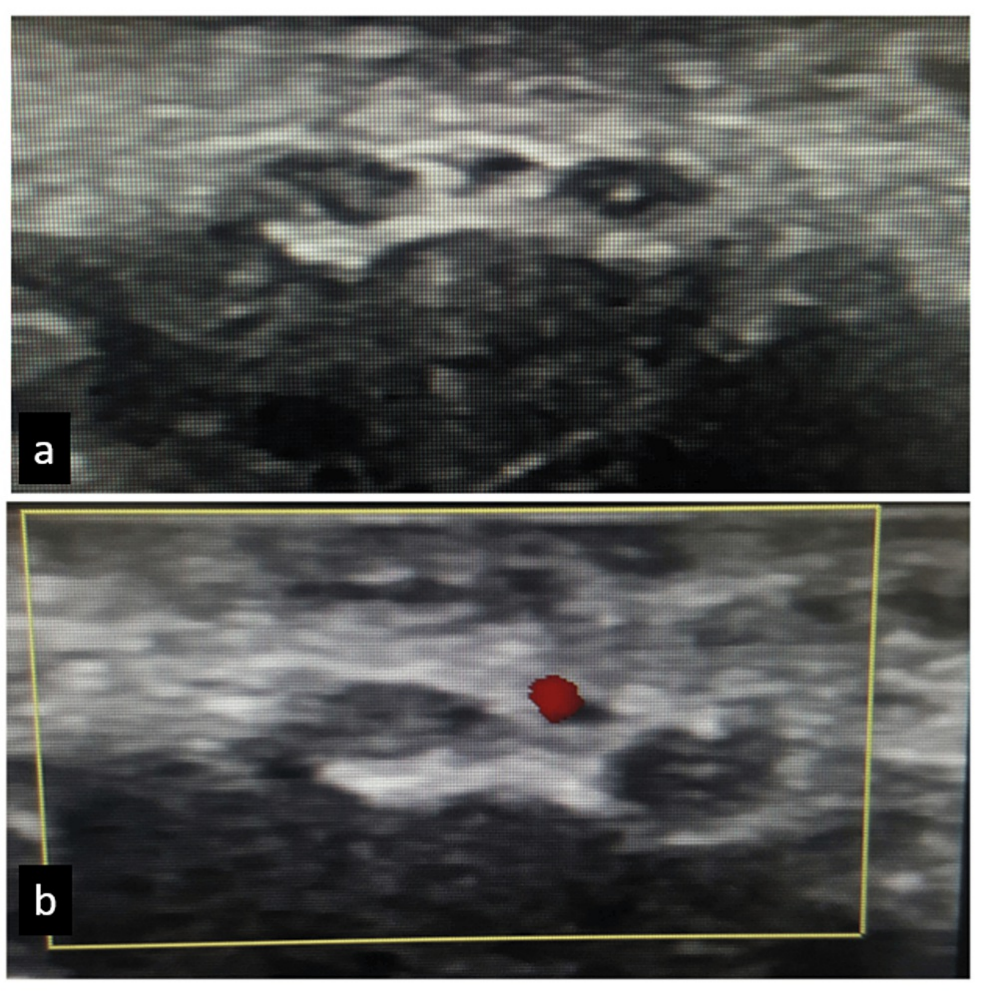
(a) B-mode and (b) color Doppler images of transverse ultrasonography of the right wrist showing the bifid median nerve and the persistent median artery
